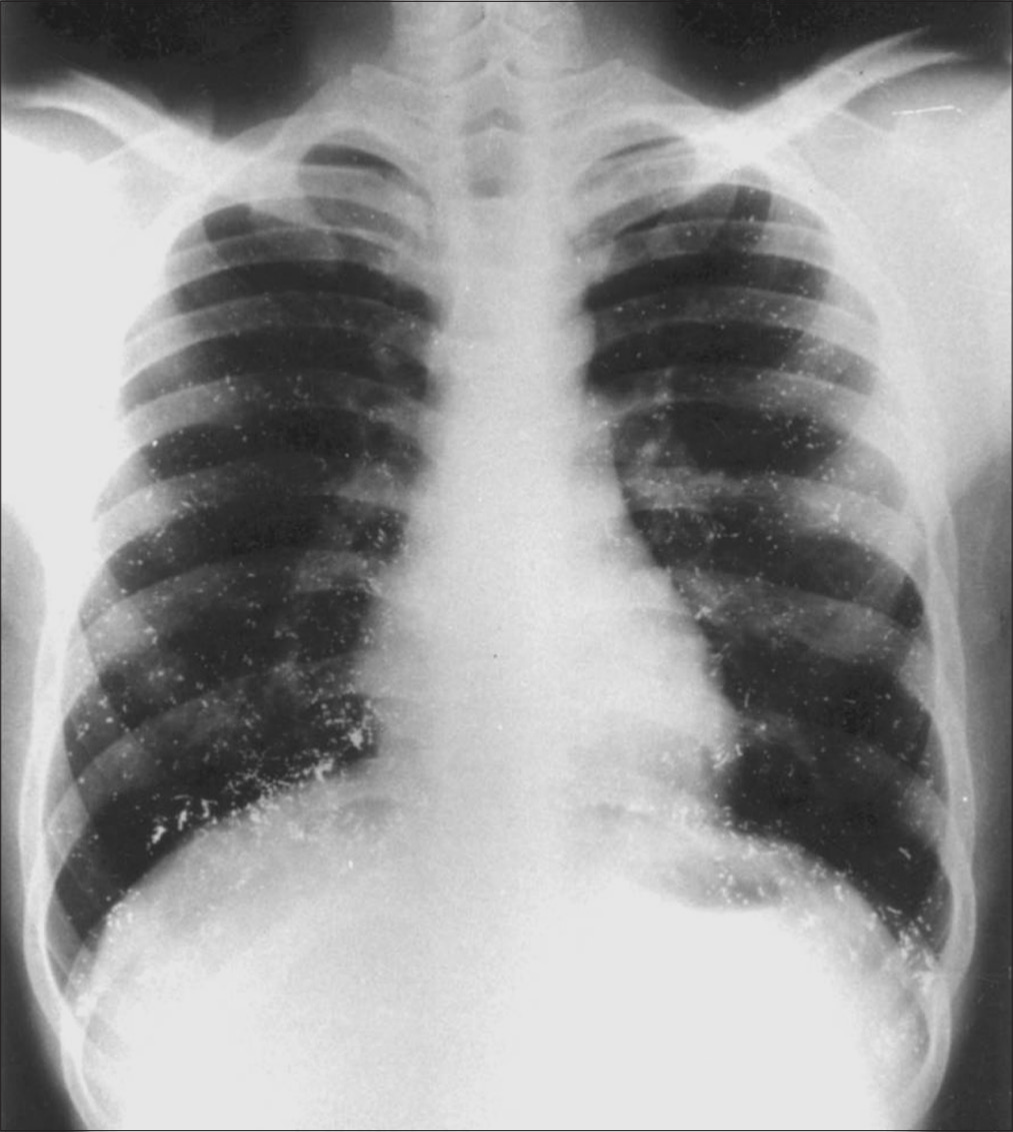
Follow-up radiograph of the chest after 6 weeks shows minimal regression of findings
